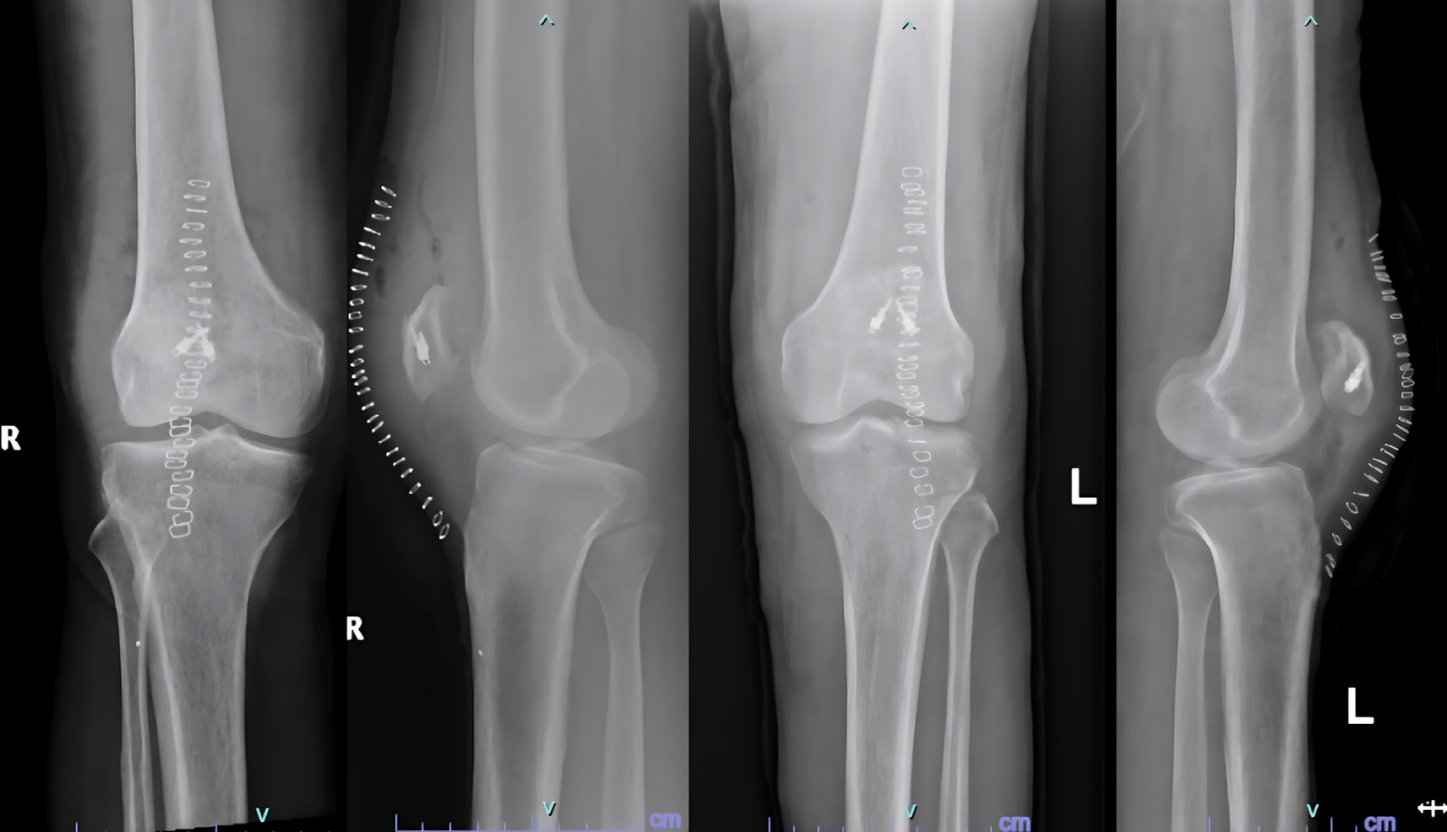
Post operative radiographs after fixation of tendons with suture anchors.
